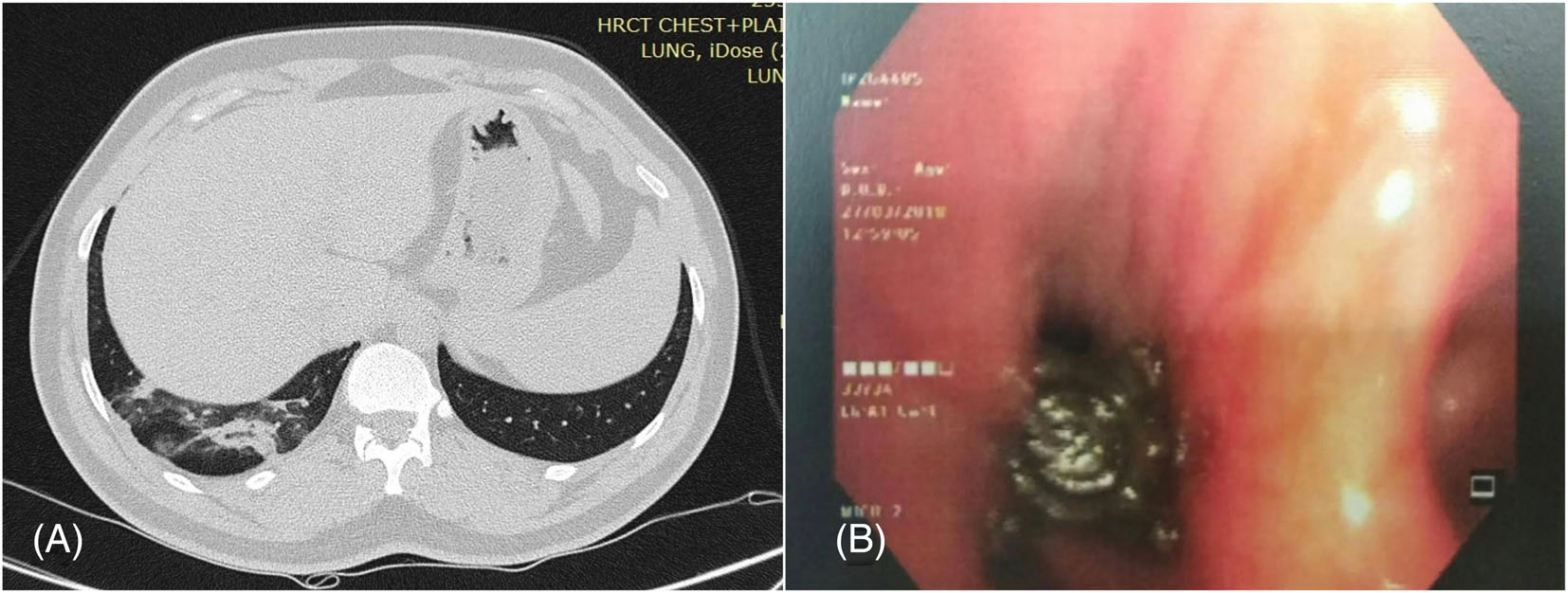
(A) Axial CT showing right lower lobe patchy consolidation and (B) foreign body in the right lower lobe medial basal segment
